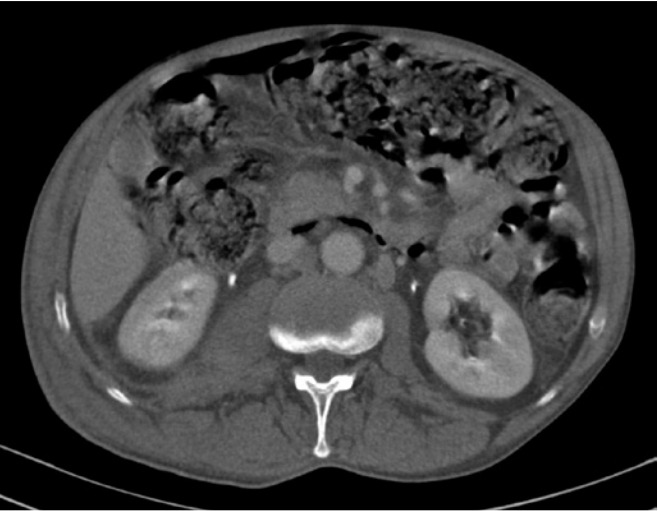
Another computed tomography slice showing pneumatosis intestinalis
